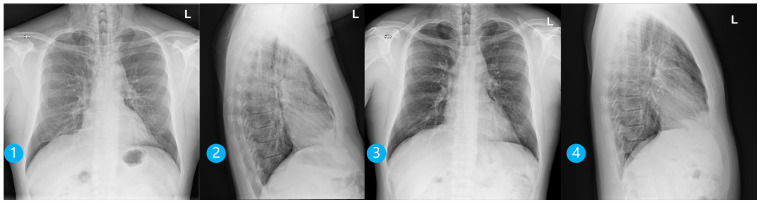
Posteroanterior and lateral chest radiographs taken on the day of emphysema occurrence (1,2) and five days later (3,4). In (1,2), subcutaneous emphysema of the soft tissues of the neck and upper thorax bilaterally is observed, along with pneumomediastinum. In (3,4), a regression of subcutaneous emphysema in the soft tissues of the neck and upper thorax bilaterally, along with pneumomediastinum, can be observed.
